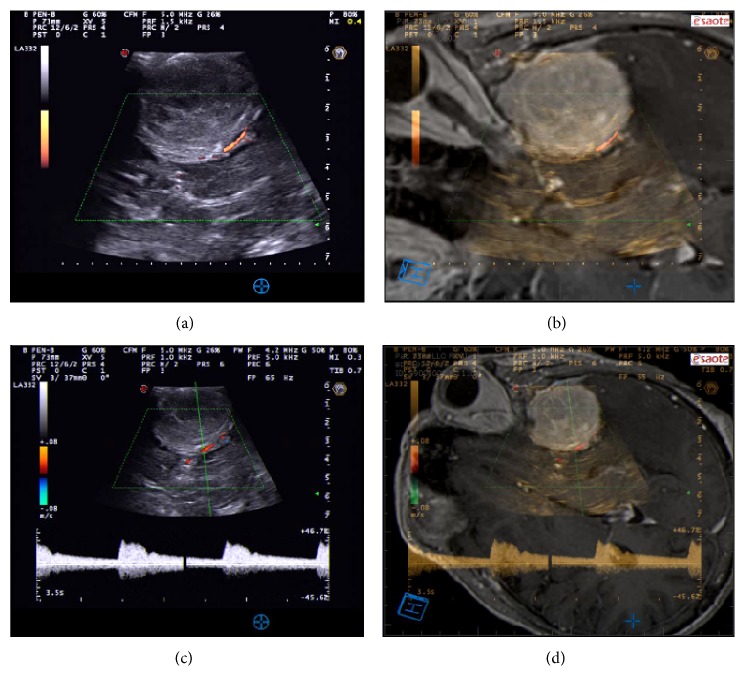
Doppler imaging. In the upper panel, power Doppler scan depicts the relationship between tumor and middle cerebral artery; this technique is less sensible to insonation angle but cannot represent flow direction and velocity. In the lower panel, color Doppler and spectral Doppler bring information about flow direction and velocity with quantification of the velocity through spectral Doppler. In the right boxes, fusion imaging between ioUS Doppler scan and corresponding preoperative MRI is displayed.
